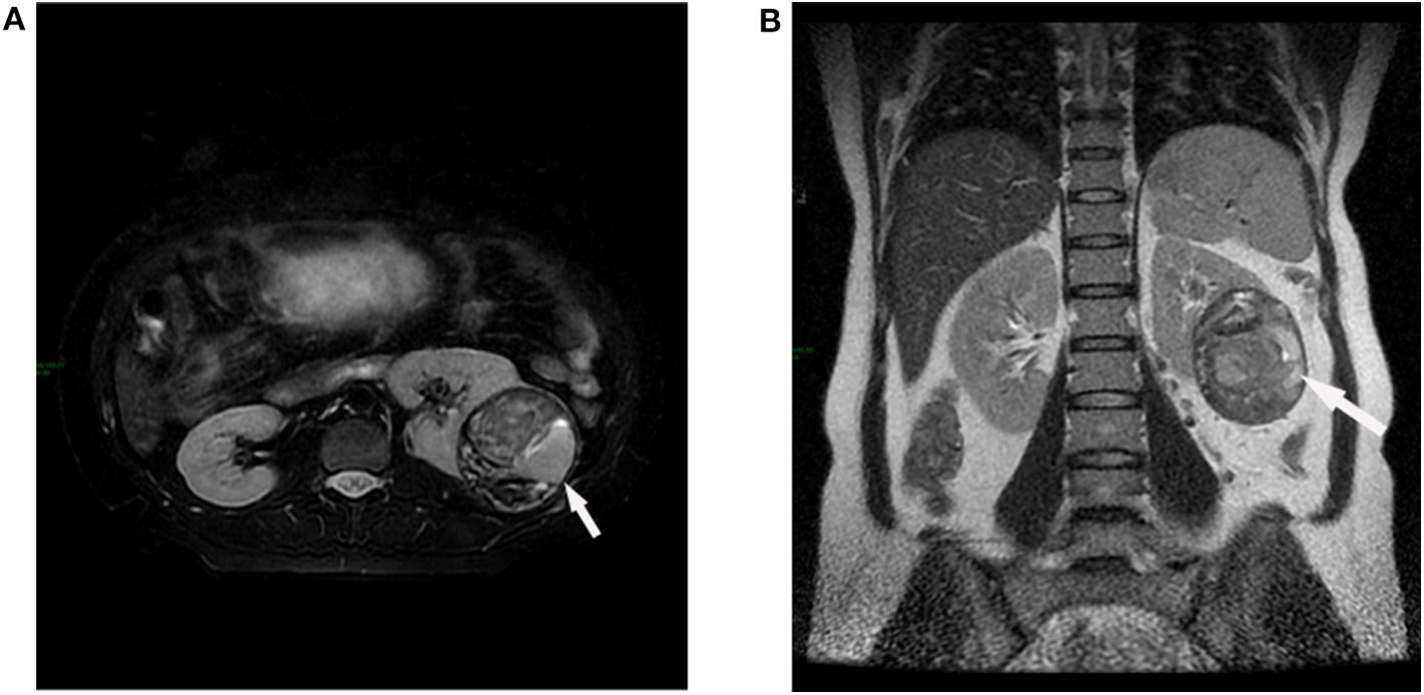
Abdominal magnetic resonance imaging (MRI). A round mass (white arrow) is shown in the middle part of the left kidney with a maximum diameter of 8.3 cm, heterogeneous signal with multiple partitions. (A) Axial plane. (B) Coronal plane.
